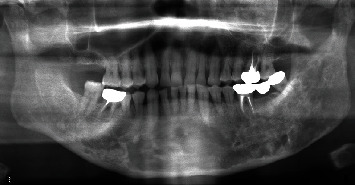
A panoramic radiograph obtained during the initial visit.
